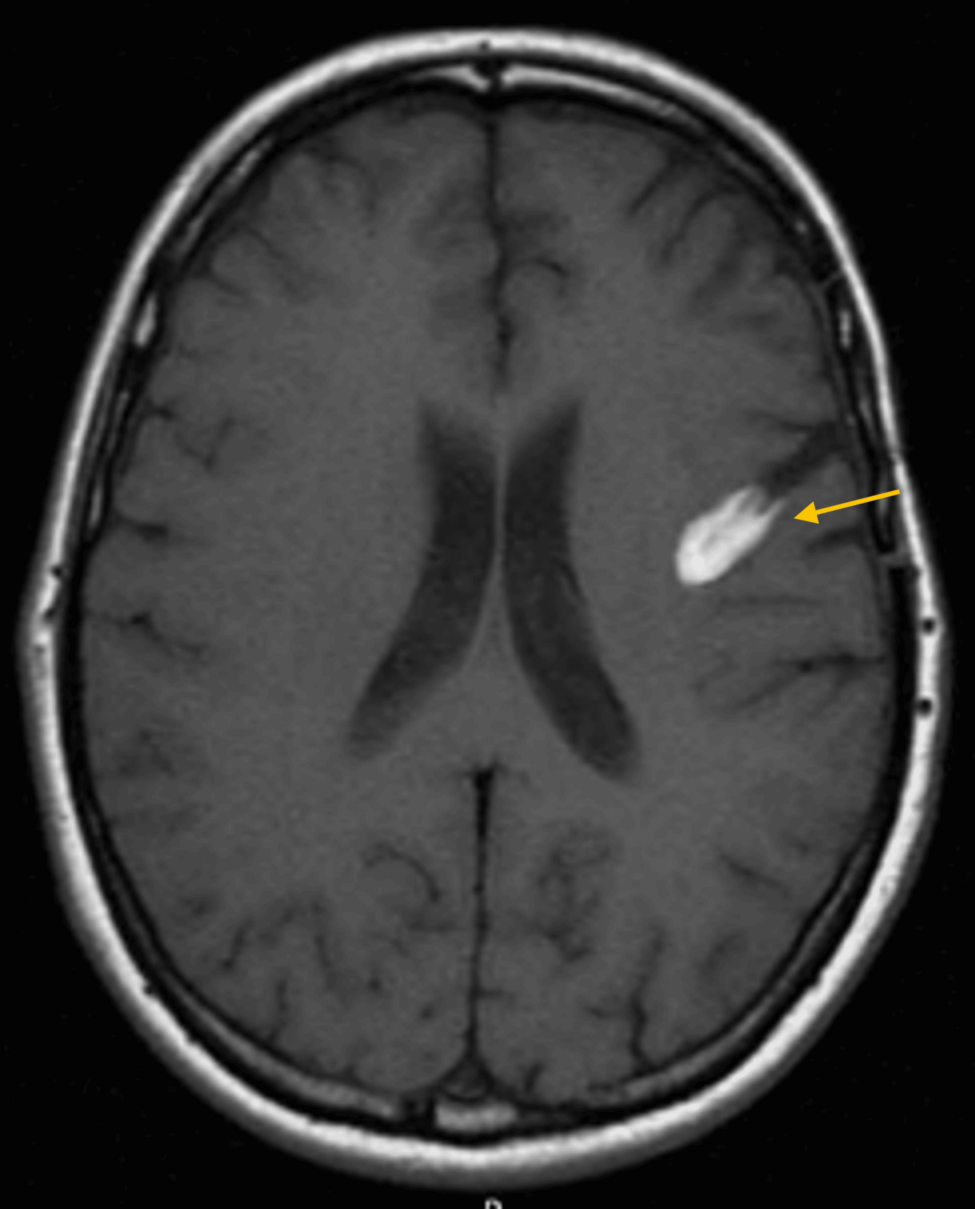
Six-month post-operative MRI The yellow arrow highlights a hyperintense T1-weighted signal at the surgical site, likely related to blood degradation products, without evidence of contrast-enhancing residual or recurrent tumor.
